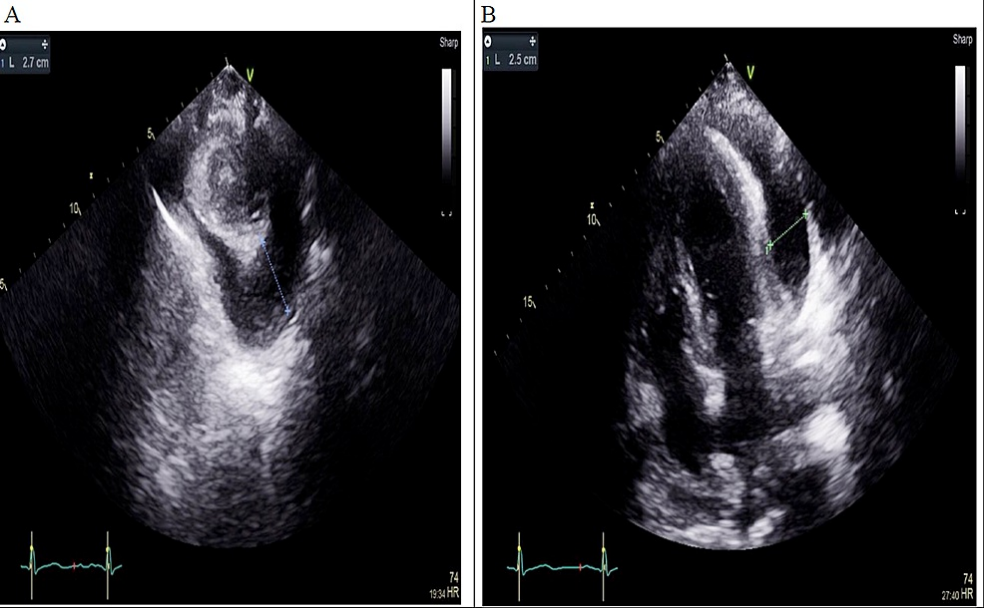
Parasternal short axis window (A) and apical 4 chamber window show large pericardial effusion (B).
